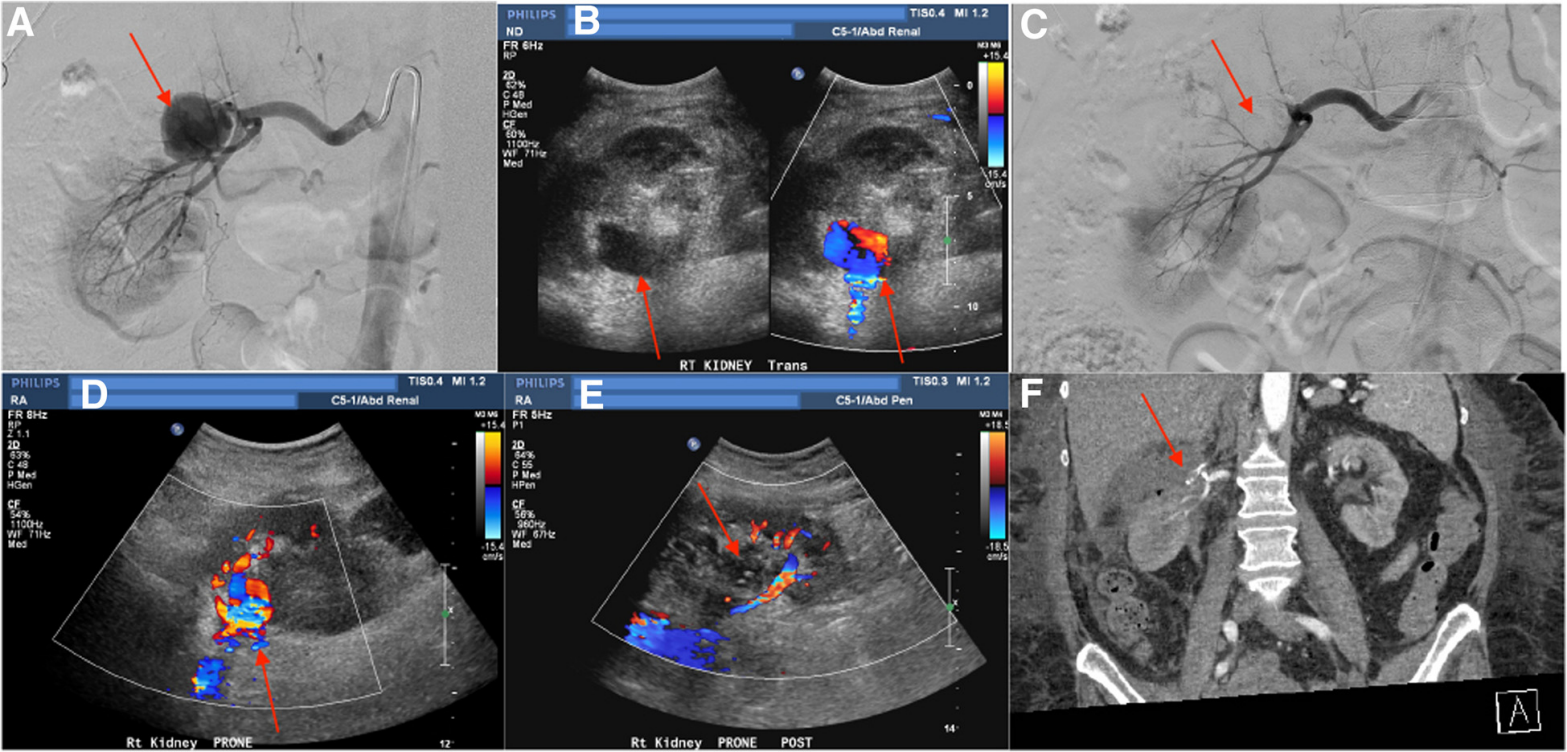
a Right renal artery angiogram demonstrates a large pseudoaneurysm arising from an interpolar branch of the right renal artery. b Renal sonography with color Doppler performed 3 days following glue embolization demonstrates persistent flow to the pseudoaneurysm. c Repeat renal angiography fails to demonstrate the residual pseudoaneurysm.d and e Color Doppler images of the right kidney before and following administration of 200 units of thrombin confirm no vascular flow in the pseudoaneurysm post-injection. f CTA examination performed 2 months following ultrasound-guided thrombin injection confirms occlusion of the pseudoaneurysm
